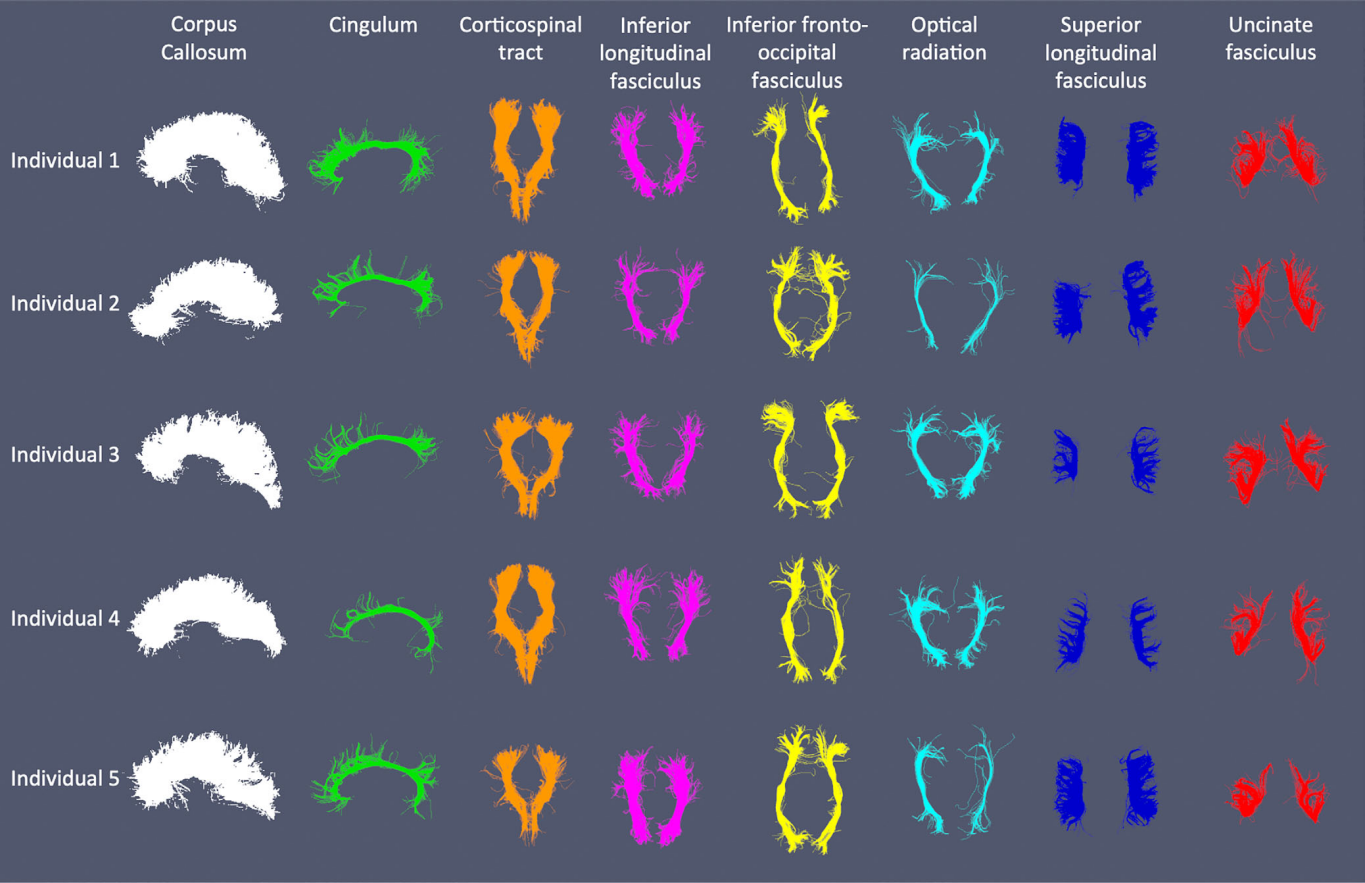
The eight white matter tracts resulting from the semiautomated tractography employed in the current study in a random selection of five of the 812 HUNT MRI individuals.
